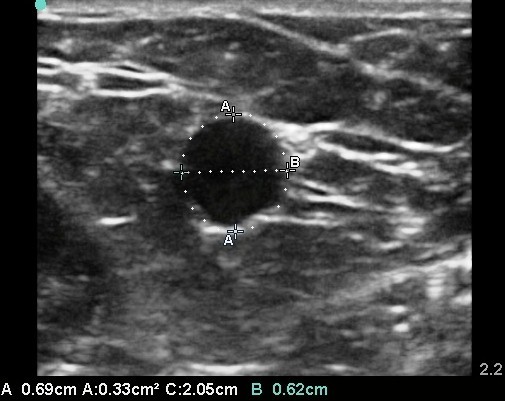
Ultrasound of basilic vein with measurement of anterior to posterior and medial to lateral diameters.
